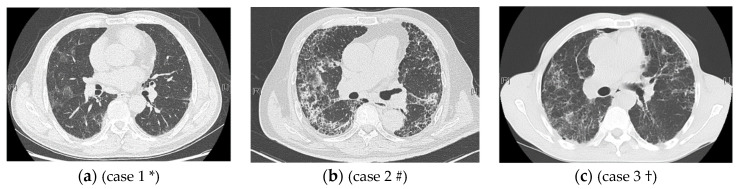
Different imaging manifestations of HRCT may affect the judgment of AI on glucocorticoid sensitivity. * Case 1 HRCT mainly shows ground glass opacity and was correctly identified as sensitive to glucocorticoids using the AI model. # Case 2 HRCT shows mainly reticulations and honeycombing and was correctly identified as insensitive to glucocorticoids using the AI model. † Case 3 is mainly characterized by ground glass opacities, reticulations, and honeycombing and was incorrectly identified as sensitive to glucocorticoids using the AI model.
